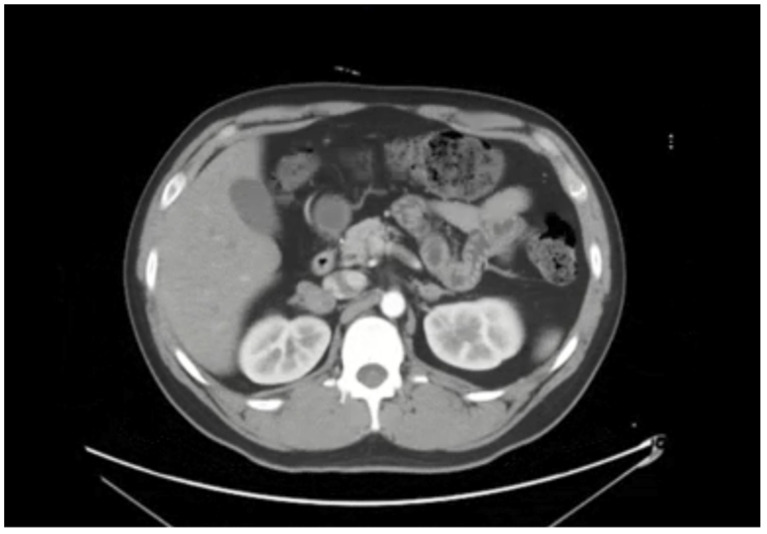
The computed tomography of the proband showed multiple nodules on both sides of the adrenal glands.
